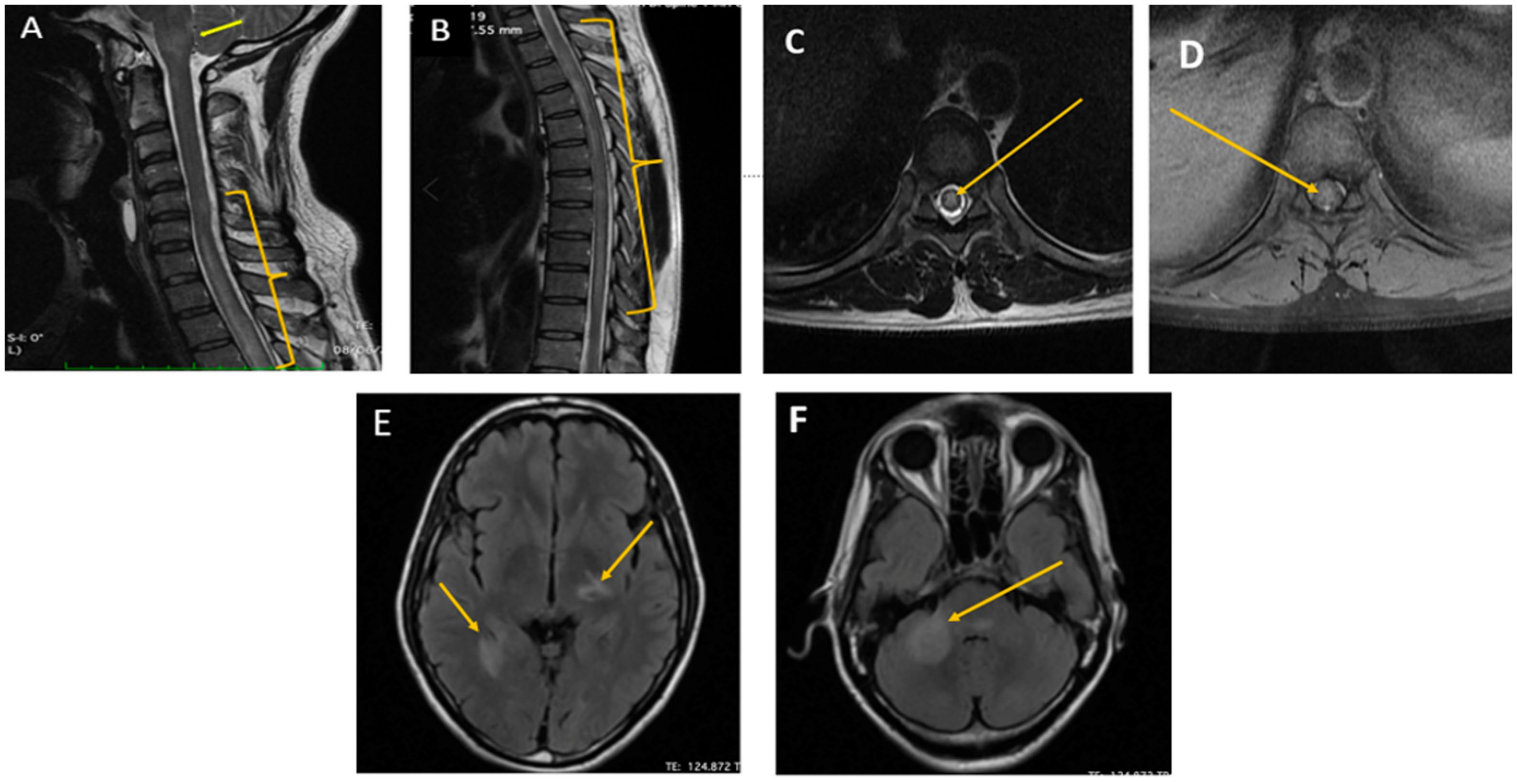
Pretreatment MRI cervicodorsal spine and brain image of the patient. (A,B) Sagittal T2/STIR images of the cervicodorsal MRI showed longitudinal extensive T2 hyperintensity in C5-D11 spinal cord segment (yellow bracket) along with brainstem involvement (yellow arrow). (C,D) Axial T2/STIR and T1 Contrast image of dorsal spine showing T2hyperintensity and partial enhancement, respectively, (Yellow arrow). (E,F) Axial FLAIR images how multiple hypointense lesions with surrounding hyperintensity in the left thalamus and the right mesial temporal and middle cerebellar peduncle suggestive of cryptococcal encephalitis (Yellow arrow).
